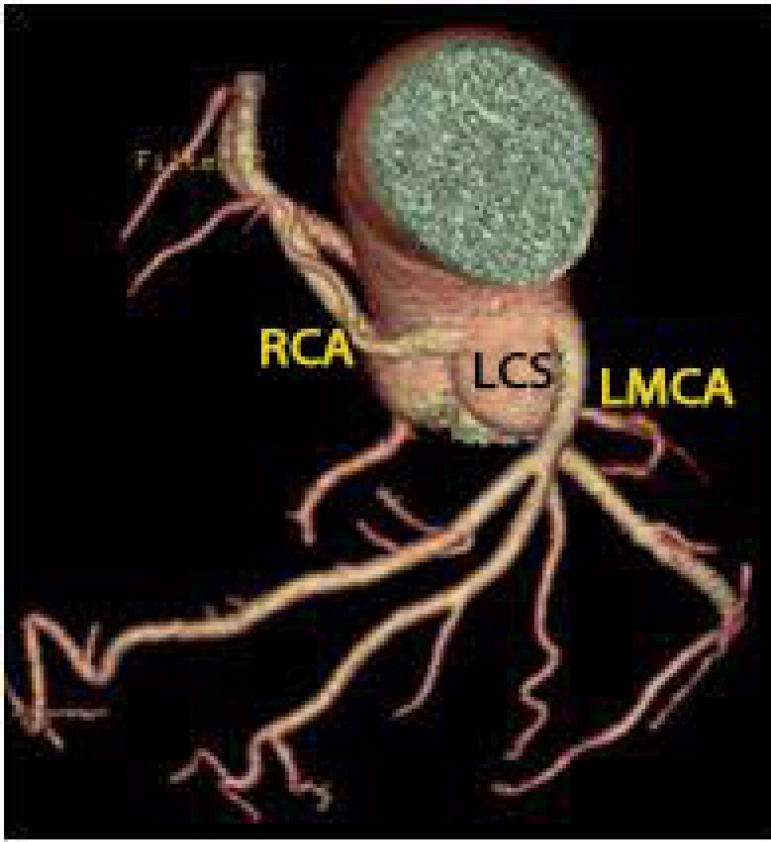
Coronary computed tomography angiography confirmed the abnormal origin of the right coronary artery from the left coronary sinus.
